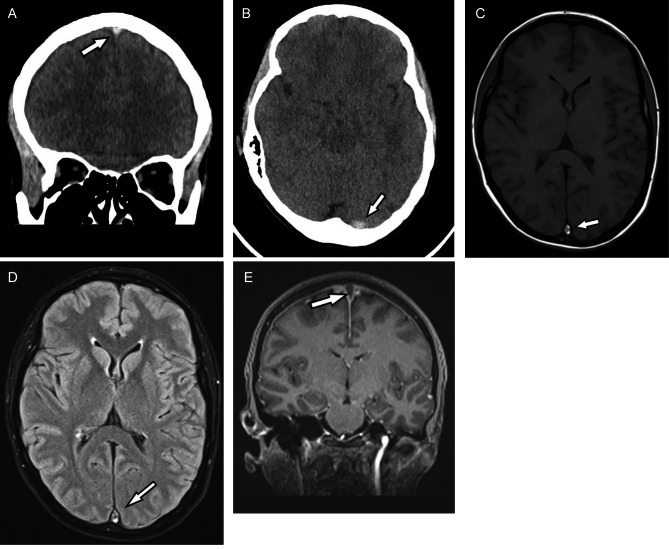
A-E. The images reveal characteristic findings of cerebral venous sinus thrombosis in a 36-year-old female patient with superior sagittal sinus thrombosis. Coronal non-enhanced CT scan (A) and axial non-enhanced CT scan (B) show areas of abnormal hyper attenuation in the superior sagittal sinus (A) and the proximal part of left transverse sinus that are consistent with thrombosis. Axial T1 weighted MRI image (C) and axial fluid-attenuated inversion recovery (FLAIR) image (D) shows thrombus as an area of increased signal intensity in the superior sagittal sinus. Coronal contrast-enhanced MR reveals thrombus as filling defects in the superior sagittal sinus (E)
